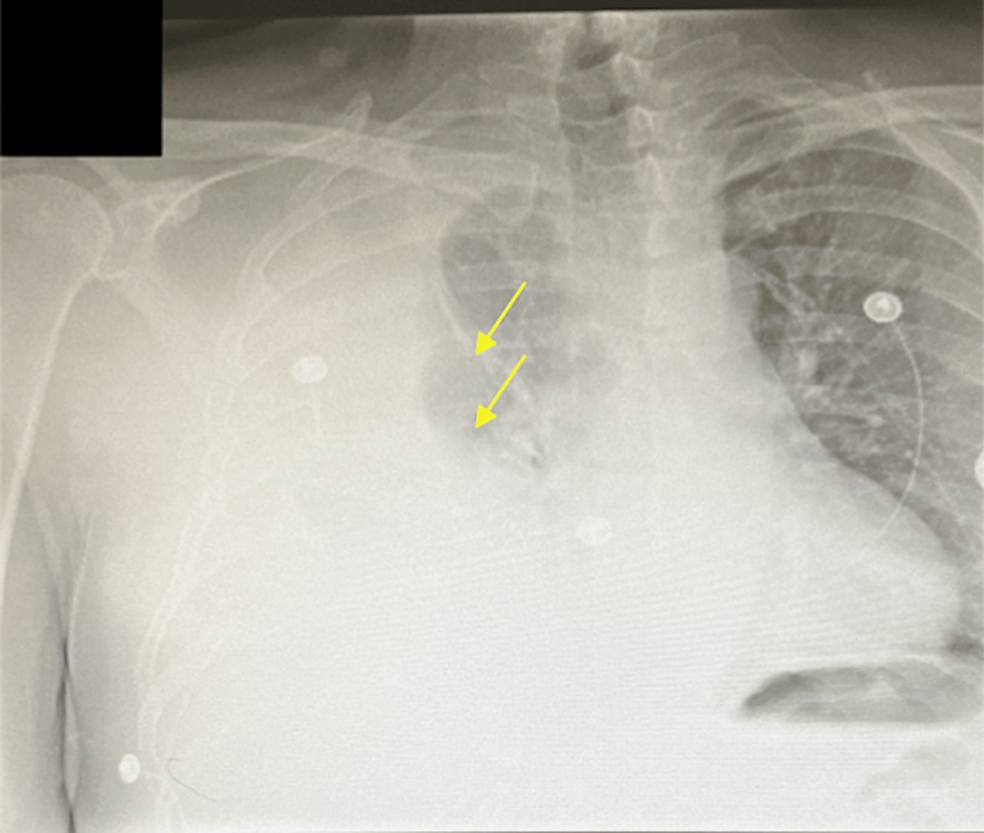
Initial chest X-ray showing pulmonary atelectasis (upper arrow) and a large pleural effusion in the right hemithorax (lower arrow)
